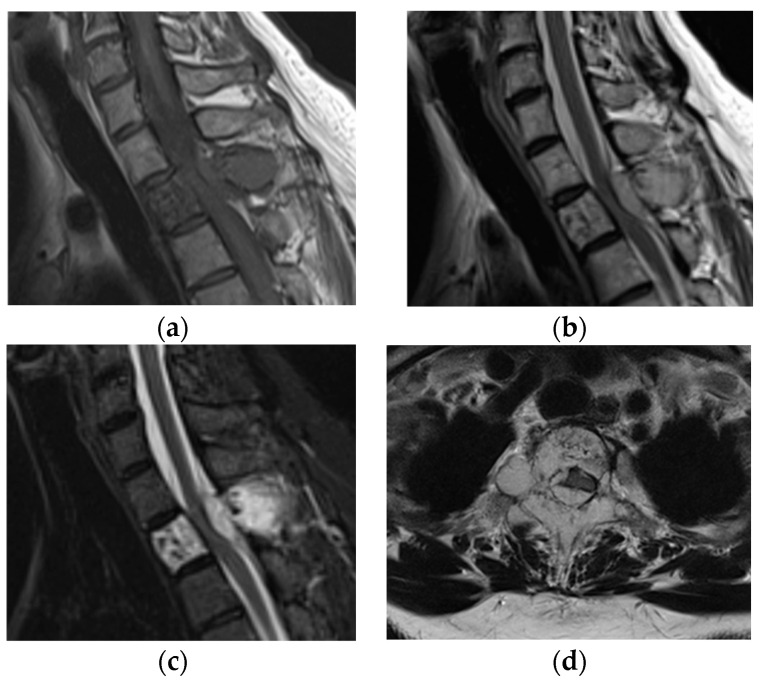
Magnetic resonance imaging of an aggressive vertebral hemangioma of the thoracic spine. A tumor with low intensity on the T1-weighted sagittal image (a) and high intensity on the T2-weighted sagittal image (b); short tau inversion recovery sagittal image (c) and T2-weighted axial image (d) compressed the spinal cord. The area had an extra-osseous region on the right.
